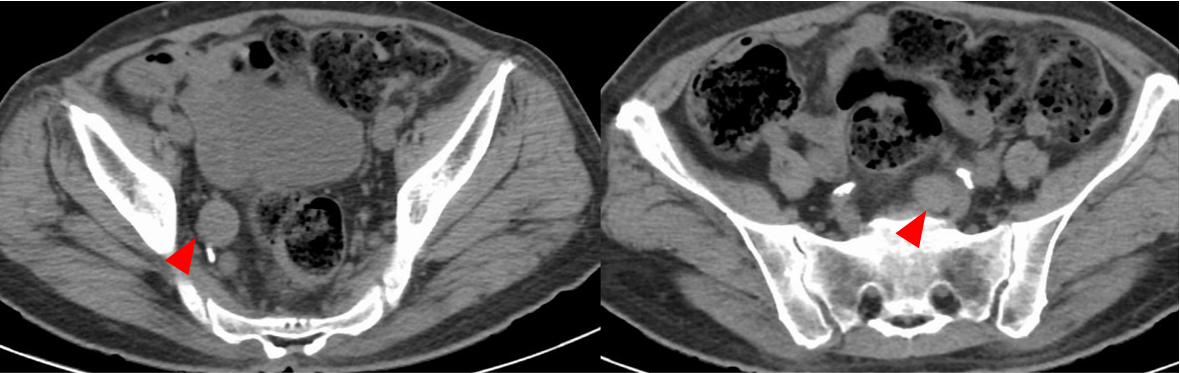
CT image of the lymphadenopathy around the rectum. Arrow heads show the lymphadenopathy around the rectum
